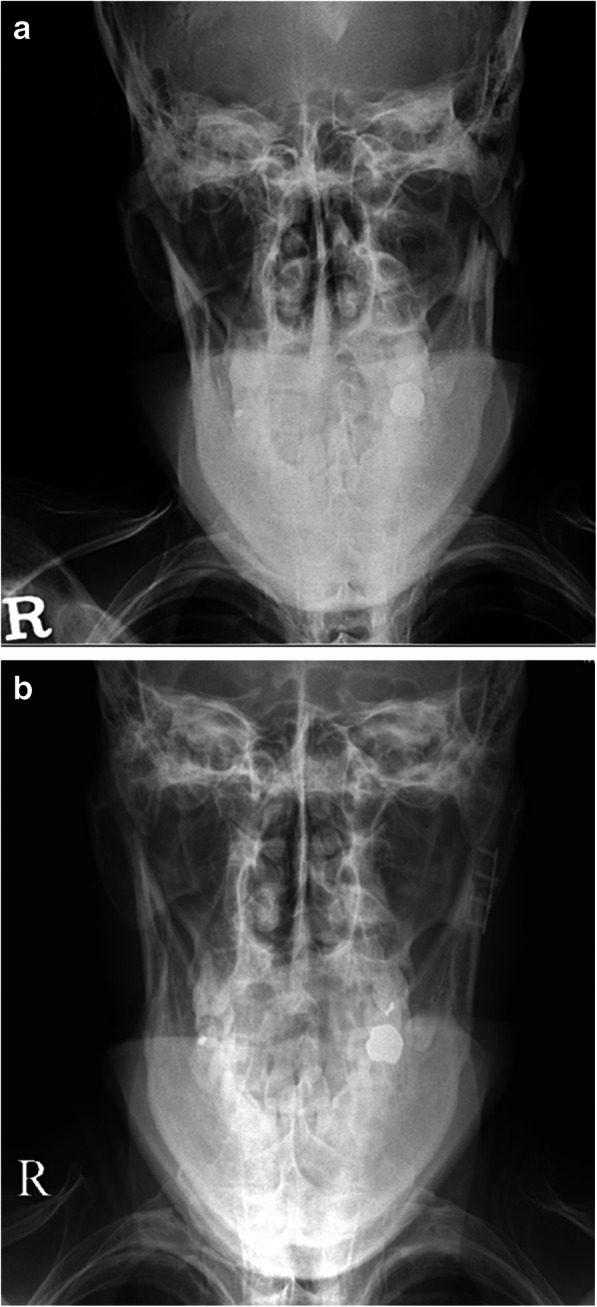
A 40 year-old male with a left subcondylar fracture of the mandible was treated using the retromandibular approach. Modified Towne’s radiographic images show the medially deviated subcondylar fracture (a) and the postoperative reduction state of the fracture following rigid internal fixation (b)
